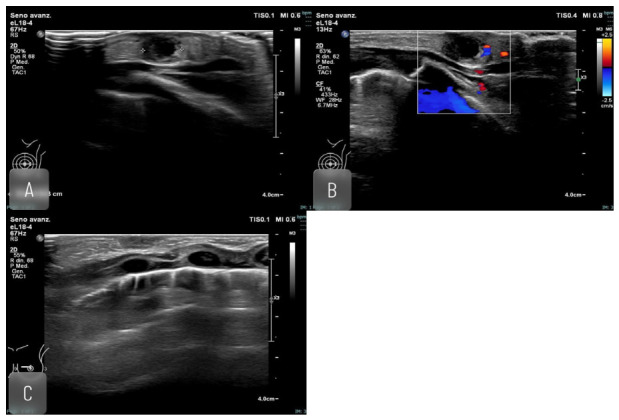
One month old newborn, with swollen left breast. US reveals pericentimetric hypoechoic breast lesion compatible with abscess. Inflammatory axillary lymph node was detected. Antibiotic therapy was administered with full recovery both clinical and ultrasound. (A,B) US imaging, breast lesion before medical therapy; (C) US imaging, breast after medical therapy.
